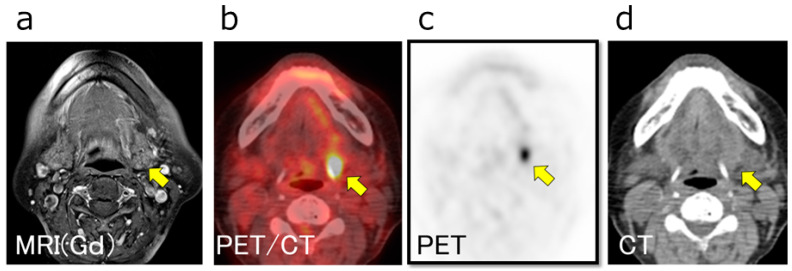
Representative case with LLN metastasis. A 79-year-old woman with a left tongue squamous cell carcinoma (SCC) (cT4aN2bM0) showed lateral lingual lymph node (LLLN) metastasis upon preoperative imaging studies. (a) Contrast-enhanced magnetic resonance imaging (MRI); (b) positron emission tomography–computed tomography (PET/CT); (c) PET; and (d) low-dose CT performed in conjunction with the PET scan. An LLLN on the left side (arrow) was not clearly detected by contrast-enhanced MRI, whereas it was clearly identified by PET/CT.
